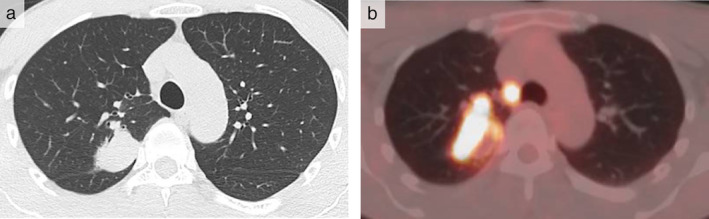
(a) Chest computed tomography (CT) scan showed a mass in the right upper lobe. (b) Positron emission tomography (PET) scan revealed a lung mass with a maximum standardized uptake value of 10.69.
